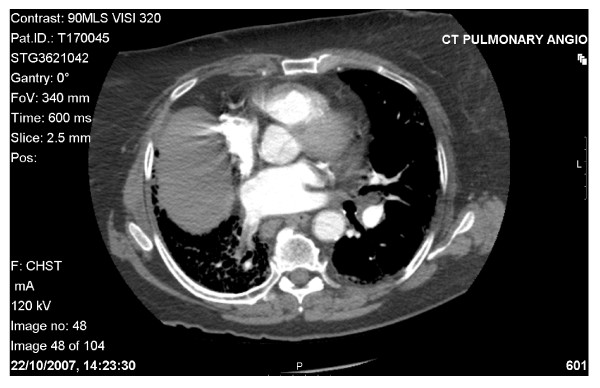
CT pulmonary arteriography with venous phase imaging. There's no evidence of pulmonary artery embolus but there is a thrombus seen in the right lower lobe pulmonary vein. There's evidence of a background of extensive right lung fibrosis and volume loss.
